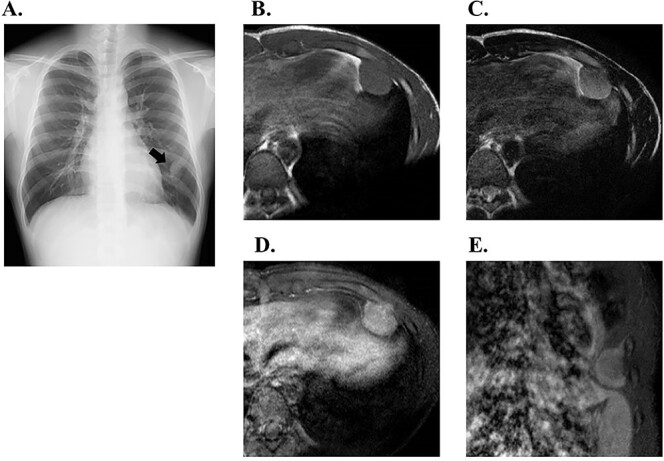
Chest X-ray of an 18-year-old male referred to our hospital (Case 4). A medical checkup in high school revealed the tumour at the left sixth rib (A, black arrow). He had no complaints, and the tumour was not palpable. Magnetic resonance imaging (MRI) revealed a 37 × 34 × 20 mm mass in contact with the pleura and located on the thoracic side. (B–E) He was diagnosed as having Ewing sarcoma by computed tomography-guided biopsy. A total of 29 days after his first visit to our hospital, he started to undergo neoadjuvant chemotherapy, surgery and adjuvant chemotherapy. At the last follow-up, he achieved a continuous disease-free status. Axial view MRI: T1-weighted image (B), T2-weighted image (C), Fat-suppressed contrast-enhanced T1-weighted image (D), coronal view MRI: Fat-suppressed contrast-enhanced T1-weighted image (E).
